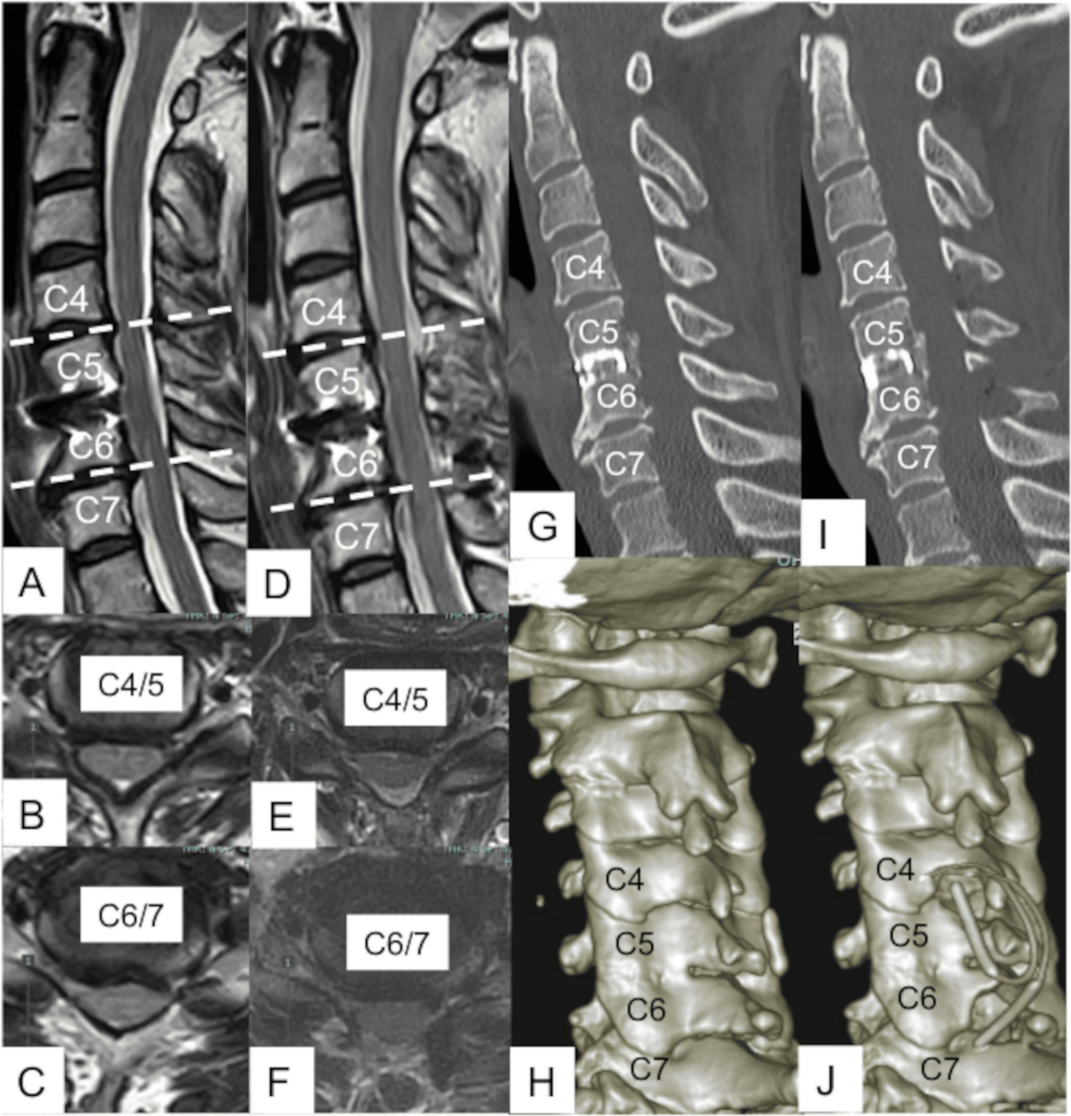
Pre- and postoperative radiological findings of Case 2. A-C: Preoperative magnetic resonance images (MRI) show C4/5 and C6/7 cord compression due to spinal canal stenosis and disc herniation (A: sagittal image, B, C: axial image). D-F: Postoperative MRIs show C4/5 and C6/7 decompression (D: sagittal image, E, F: axial image). G, H: Preoperative computed tomography images show C5/6 anterior fusion (G, a sagittal image, H: three-dimensional reconstructed image). I, J: Postoperative CT images show slip laminectomy at C4/5 and C6/7 (I, a sagittal image, J: three-dimensional reconstructed image).
